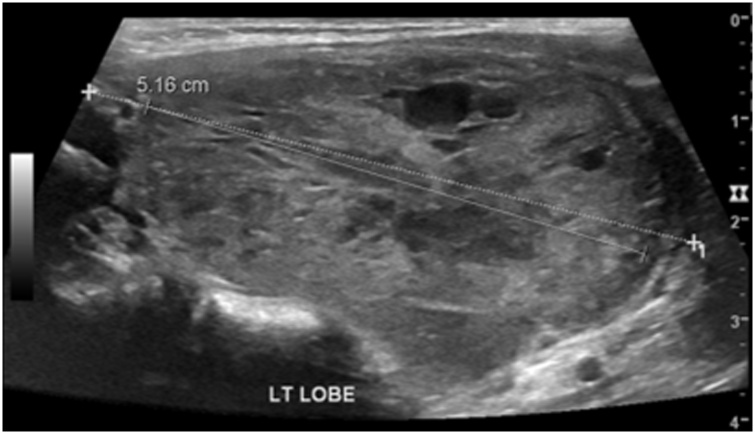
Thyroid ultrasound showing a nodule in the left lobe of the thyroid gland.
